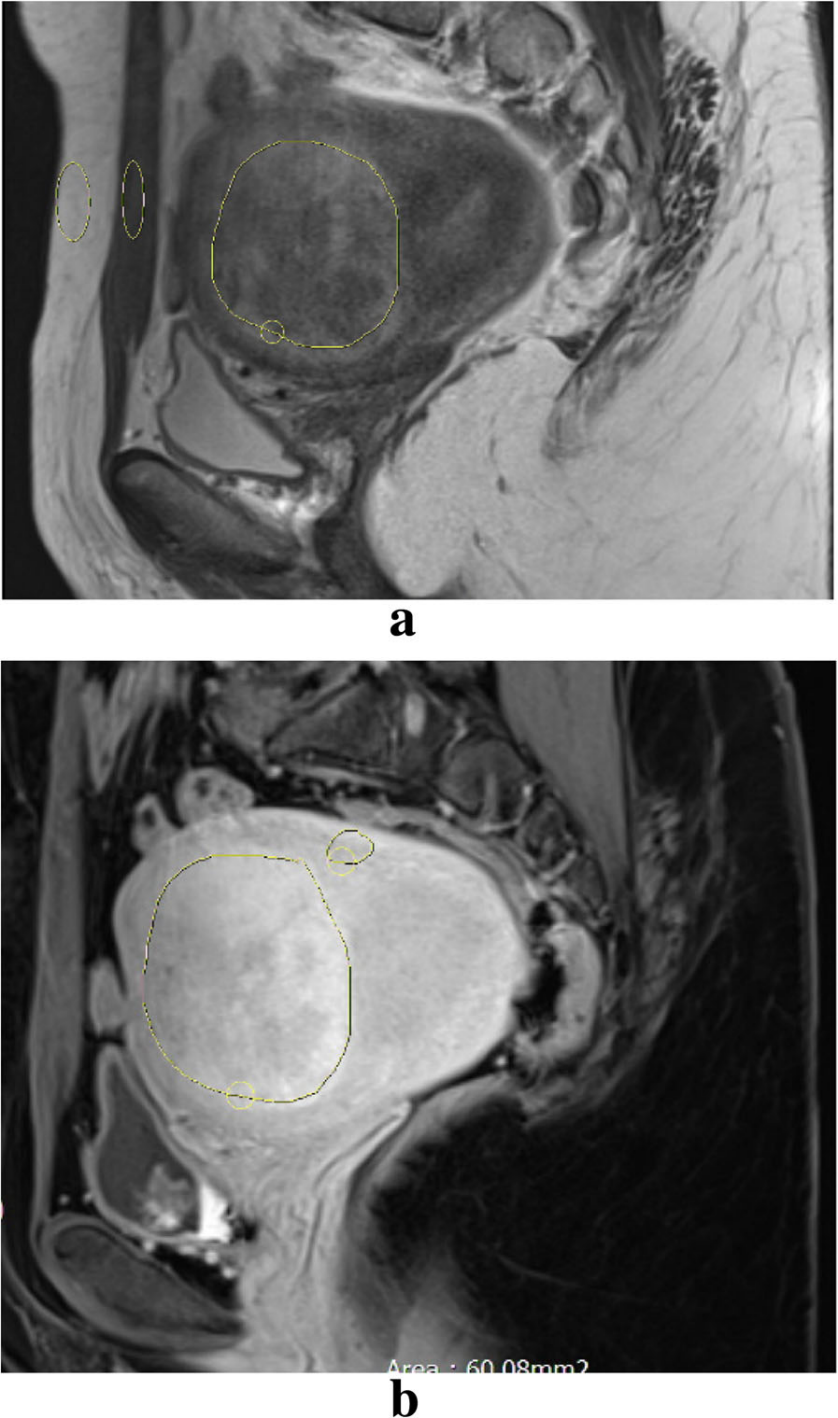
A 22-year-old patient with history of AUB and cellular leiomyoma on pathology. a A round high signal myometrial lesion on sagittal T2-weighted image, T2 scaled ratio = 0.28. b Sagittal contrast enhanced T1-weighted image with tumor psoas contrast enhanced ratio = 1.100
